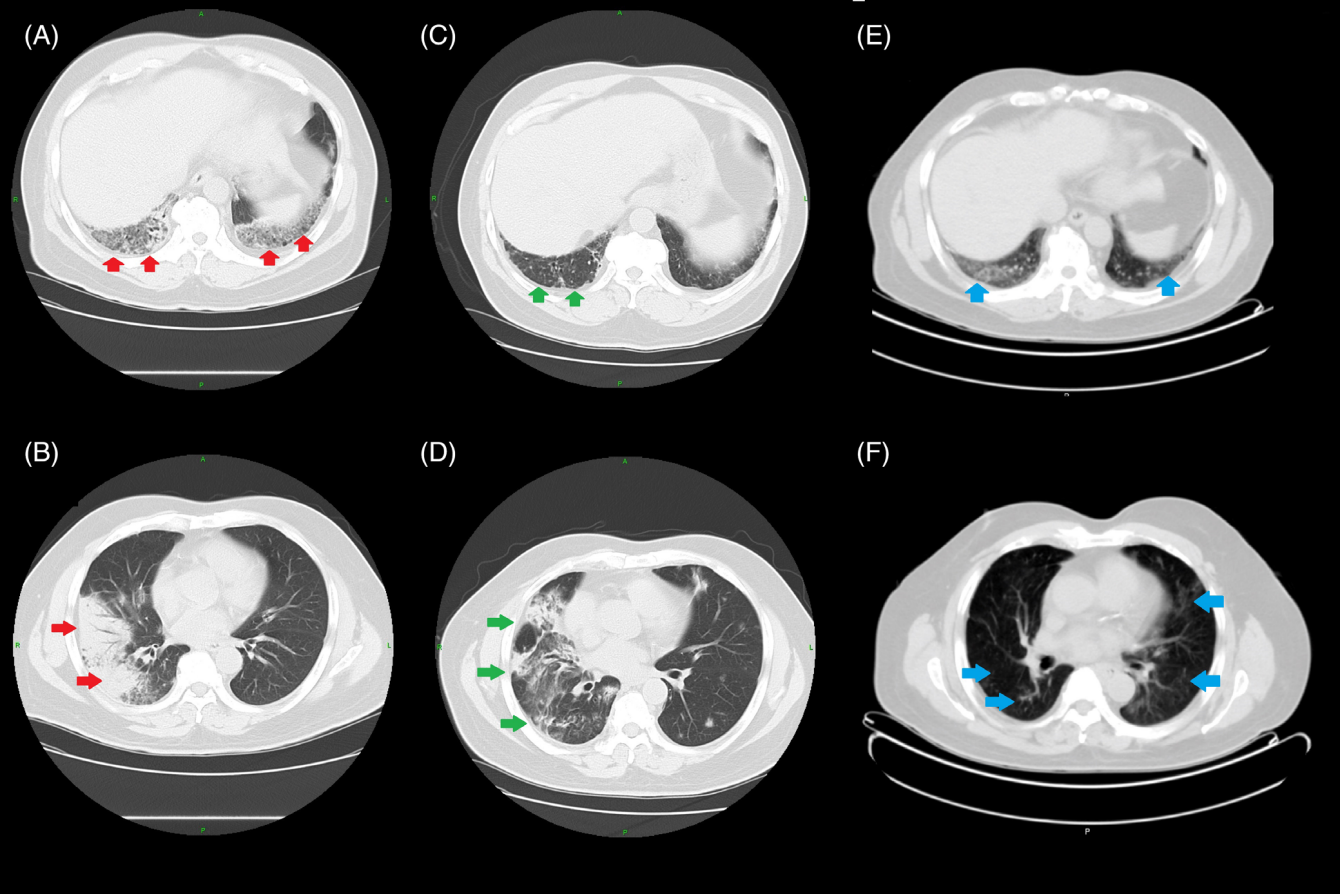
(A, B) CT scan in February 2021: Focal consolidative airspace disease in the posterior lateral right lower lobe and posterior basilar left lower lobe. Multifocal, peripheral ground‐glass opacities with round morphology more numerous in the right lung. Features are consistent with organizing pneumonia seen with drug toxicity. (C, D) CT scan in April 2021: Improved dense consolidation in the right lower lobe but the multiple small areas of focal consolidation within the right upper lobe on the previous examination have progressed since the previous study and there are also multiple new areas of focal irregular consolidation with central air bronchograms involving both lungs representing inflammatory process. (E, F) CT scan in October 2021: Diffuse ground‐glass infiltrates bilaterally in the lungs demonstrating mild metabolic activity with SUV of 2.2 concerning for unresolved pneumonitis.
